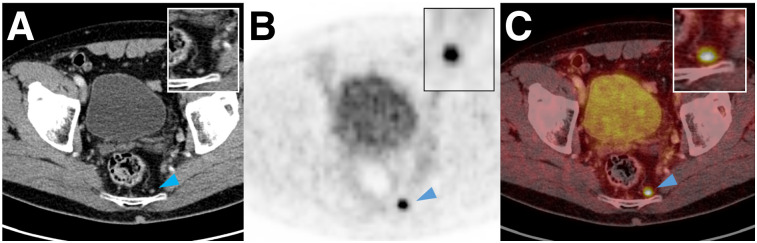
Axial CT (A), ^18^F-PSMA-1007 PET (B), and ^18^F-PSMA-1007 PET/CT (C) images of 65-y-old patient with prostate adenocarcinoma (initial PSA, 23.8 ng/mL). There is 1 positive LN (arrowhead) close to plexus sacralis that was not removed during surgery. Patient’s prostate-specific antigen level continued to rise after surgery, indicating that retained node was malignant.
